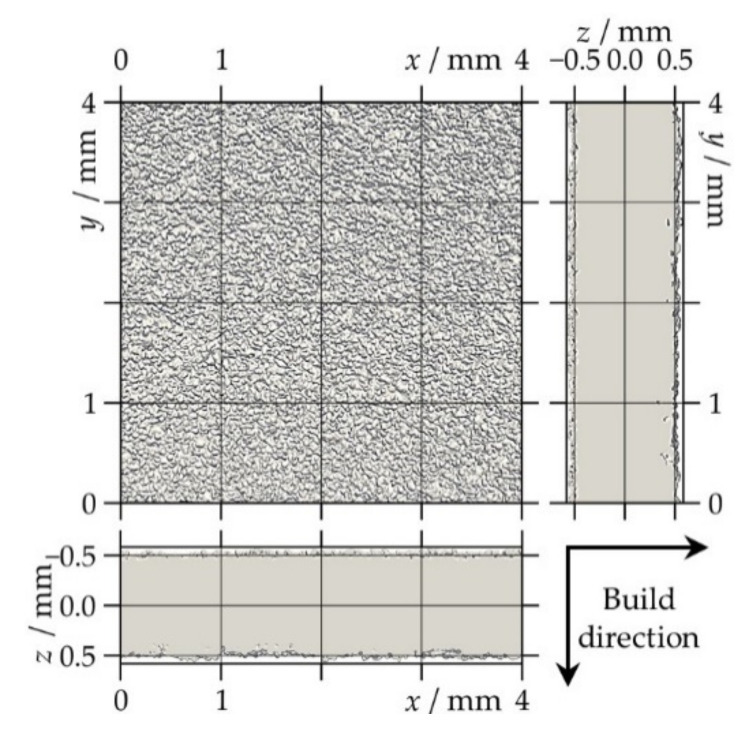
Three orthogonal views of the volume of interest for the X-ray micro-computed tomography data analysis of a laser-sintered polyamide 12 sheet generated with eight layers. The arrows indicate the build direction that corresponds to the positive *z*-direction in the two lateral views.
